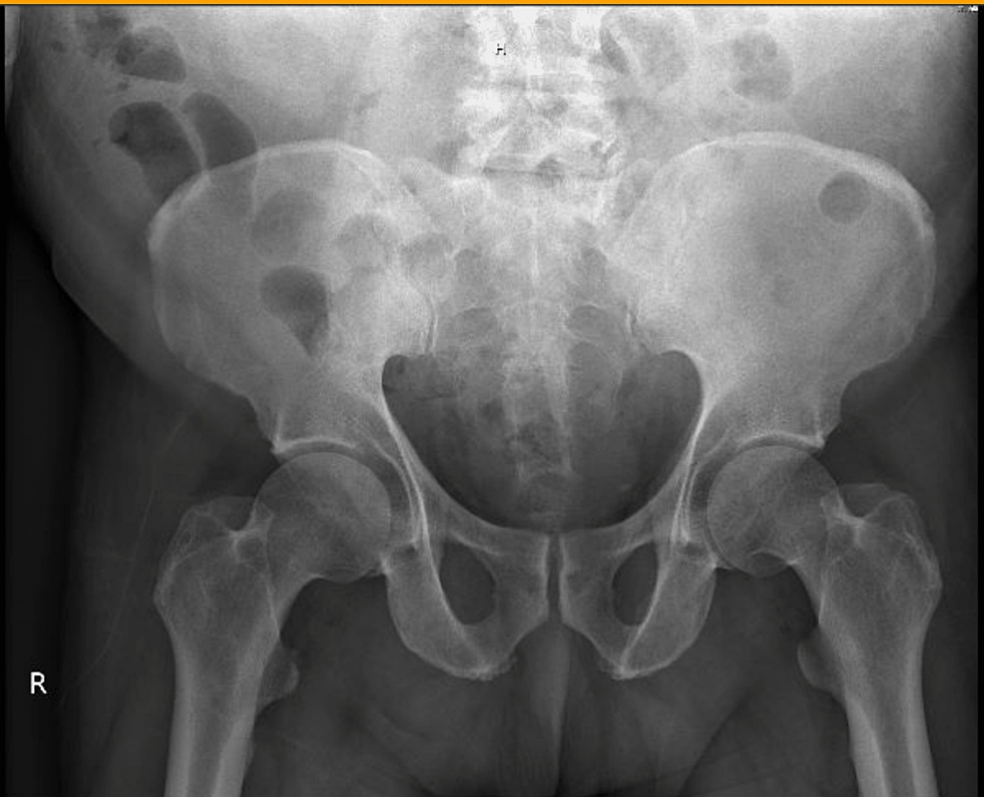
X-ray of pelvis and bilateral hips reveals normal findings
